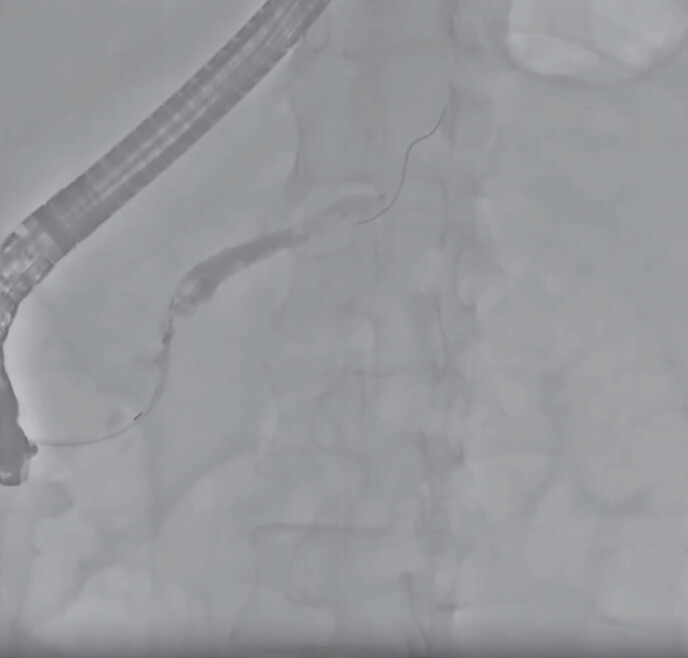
X-ray imaging showed that a basket shadow containing extensive
